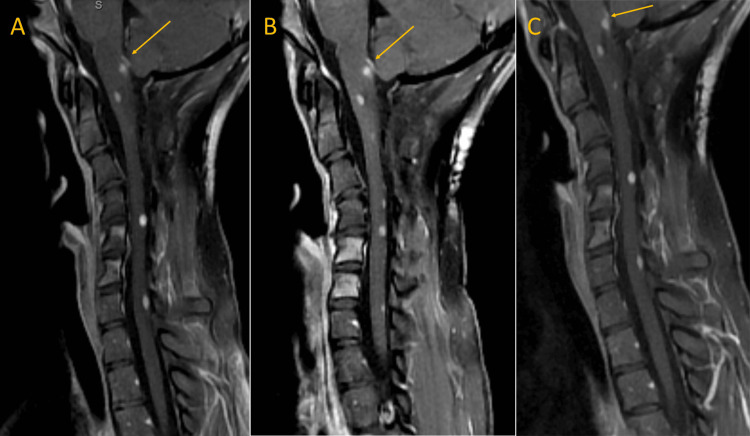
Follow-up magnetic resonance imaging (MRI) of the cervical spine at 6, 9, and 12 months, demonstrating a sustained radiologic response (T1 post-contrast) (A) Six-month cervical MRI. (B) Nine-month cervical MRI. (C) 12-month cervical MRI.
